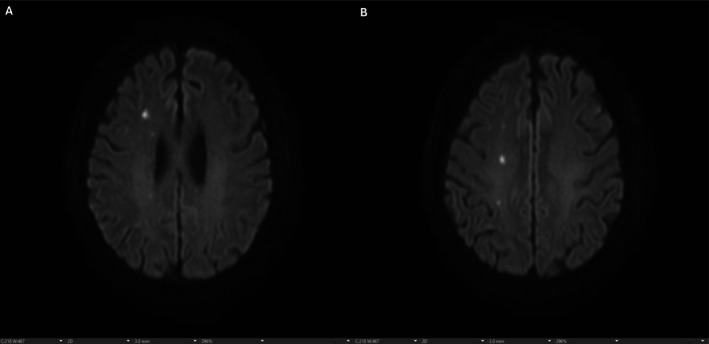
(A and B) Axial diffusion‐weighted MRI of the brain demonstrating multiple hyperintense foci within the cerebral parenchyma, consistent with septic emboli.
